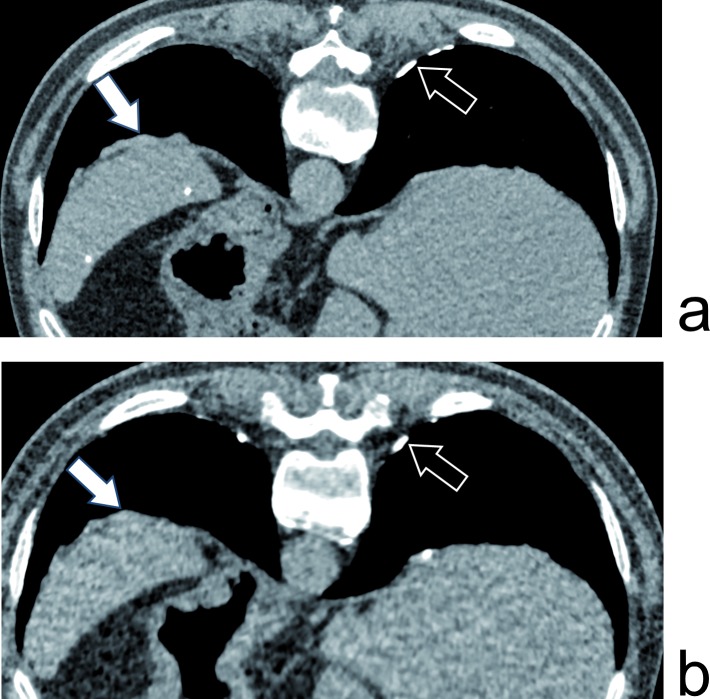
Pleural plaques. 61yo male with 19 years of asbestos exposure. Pleural plaques, calcified (black arrow) and non-calcified (white arrow) are depicted with equal efficiency by the reference full dose CT (a–DLP of 301.7mGy.cm) and the ULD acquisition (b–DLP of 19mGy.cm).
